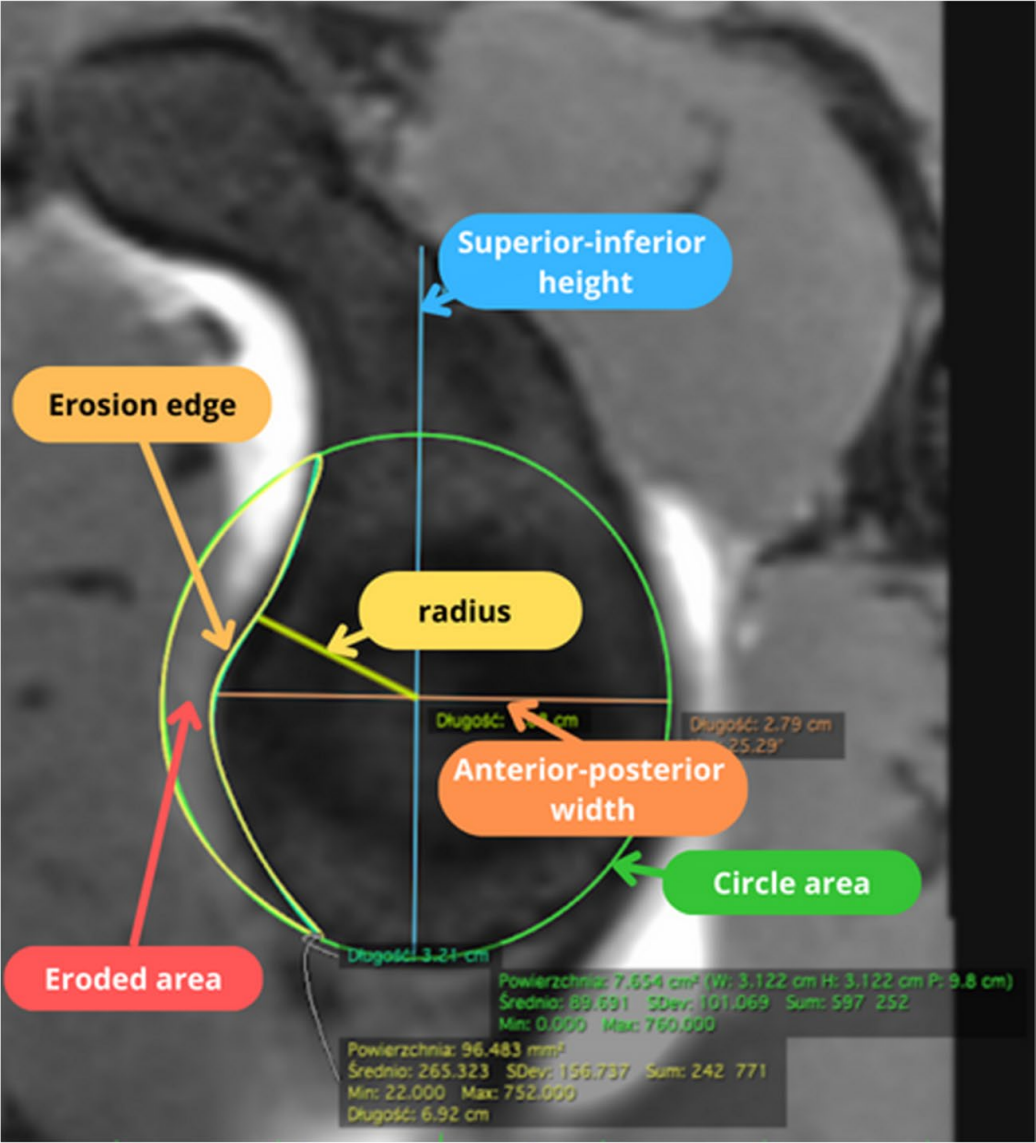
Sample measurement of glenoid parameters on MRI image - vibe sequence
